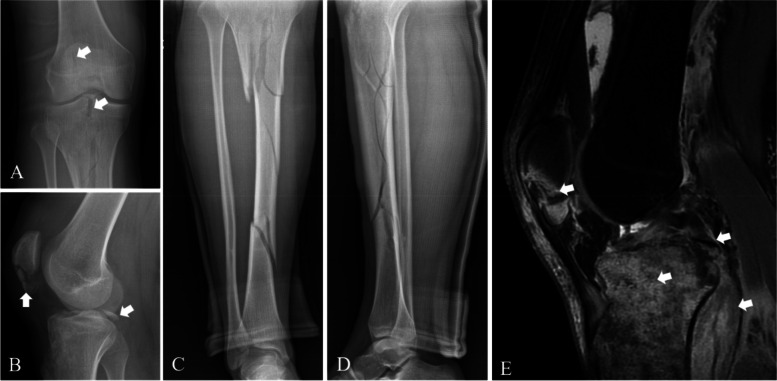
Preoperative right knee radiographs of an 18-year-old male: AP (A) and lateral (B) views showed patellar comminuted, proximal tibial comminuted and PCL tibial avulsion fractures. Preoperative tibial and fibular radiographs: AP (C) and lateral (D) views revealed multiple comminuted fractures. Preoperative sagittal fat-suppressed T2-weighted magnetic resonance imaging (MRI) of the right knee (E) demonstrated patellar and PCL tibial avulsion fractures and peri-knee soft tissue and tibial marrow oedema (white arrow)
